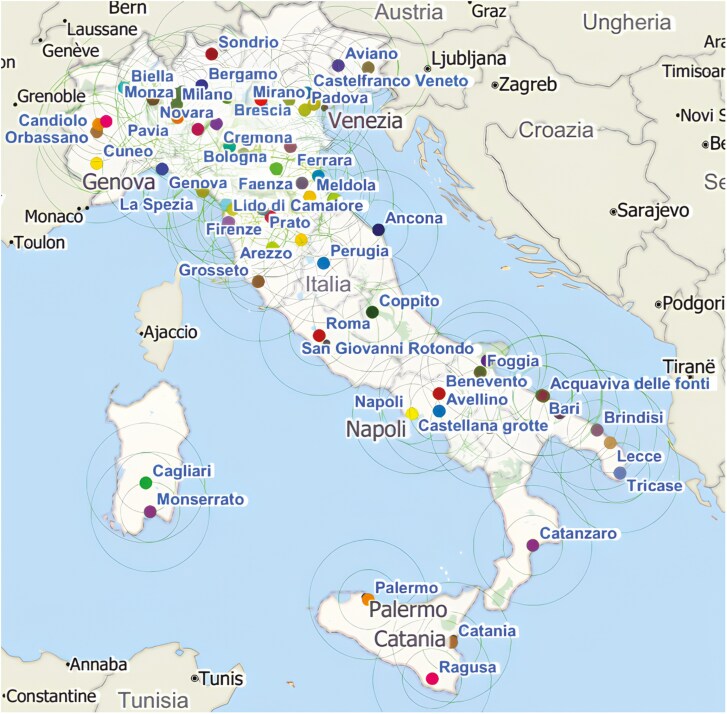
Distribution of clinical centers involved in CT in gastroenterological oncology. Areas of influence at 10, 50, and 100 km from the center are drawn for each Clinical Center.
